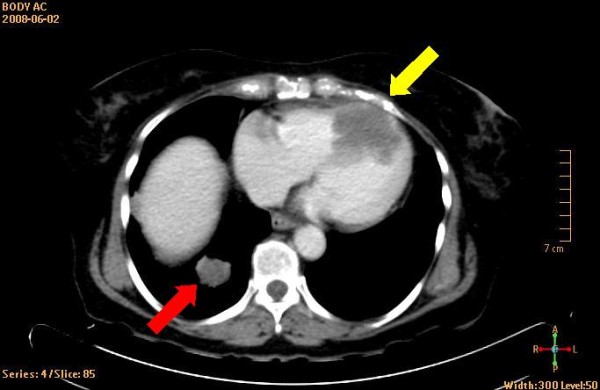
Display of CT-image. The yellow arrow indicates the metastasis of the leiomyosarcoma. The red arrow indicates of the lung metastases.
